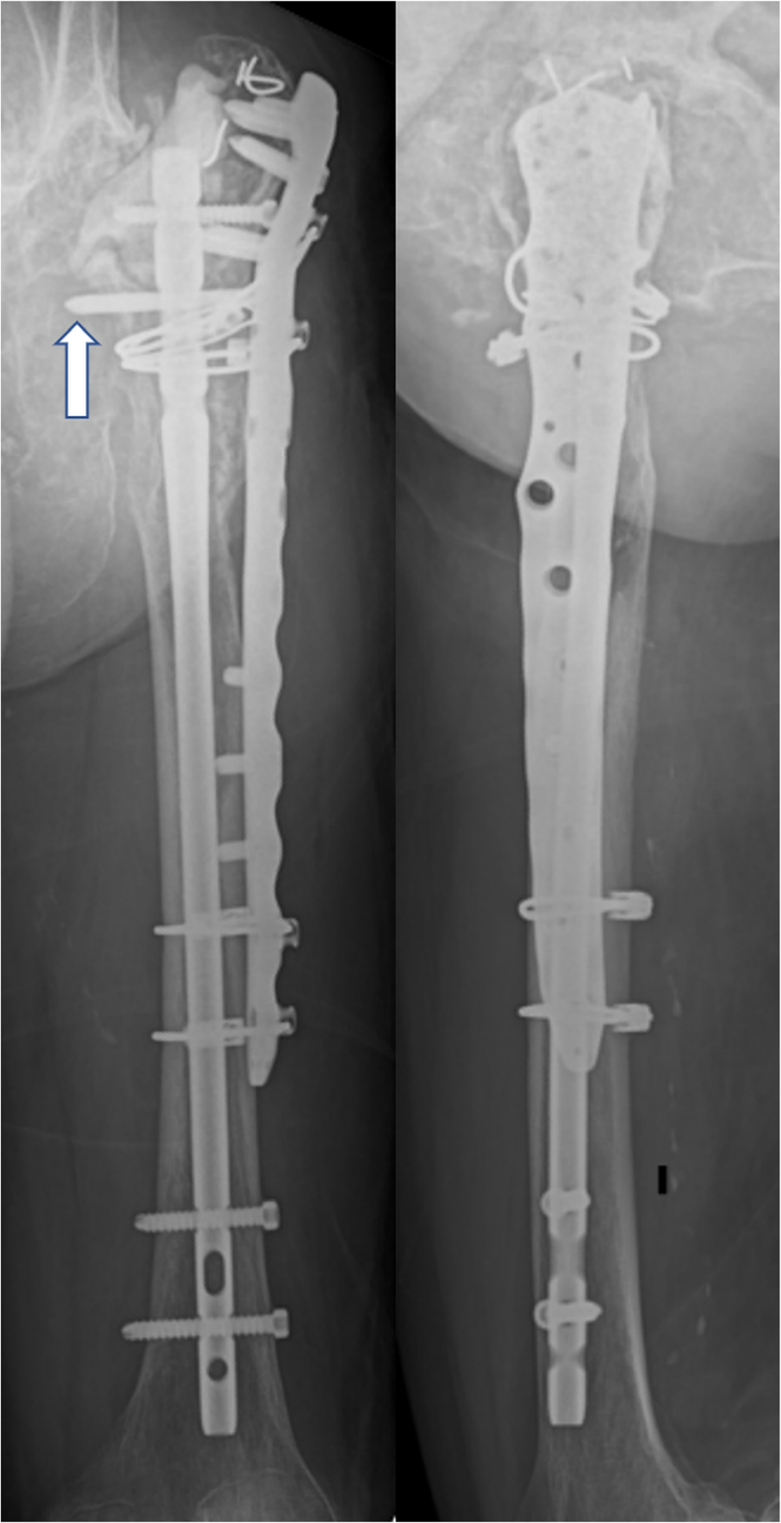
Postoperative plain radiographs of the left femur (left: anteroposterior view, right: lateral view). A locking screw of the plate was placed through the intramedullary nail to reinforce the fixation (arrow)
